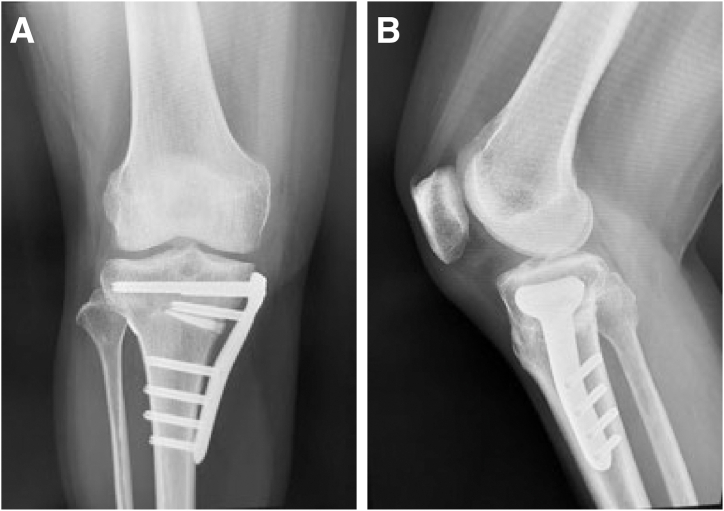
Postoperative radiographic images of right knee. (A) Frontal view. (B) Lateral view. The pullout button (AI-Medic) is secured on the lateral side of the tibia.
